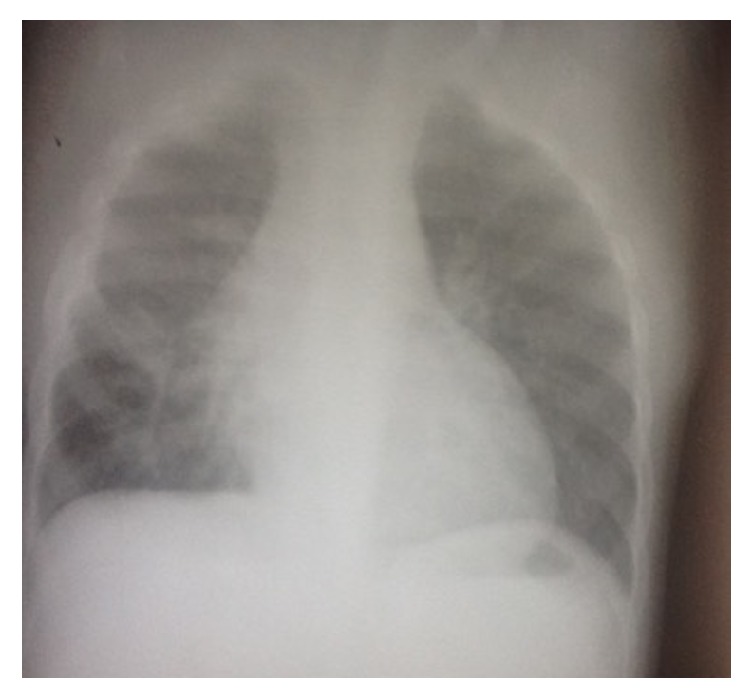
Chest X-ray, control one month after the surgery in the baby boy.
